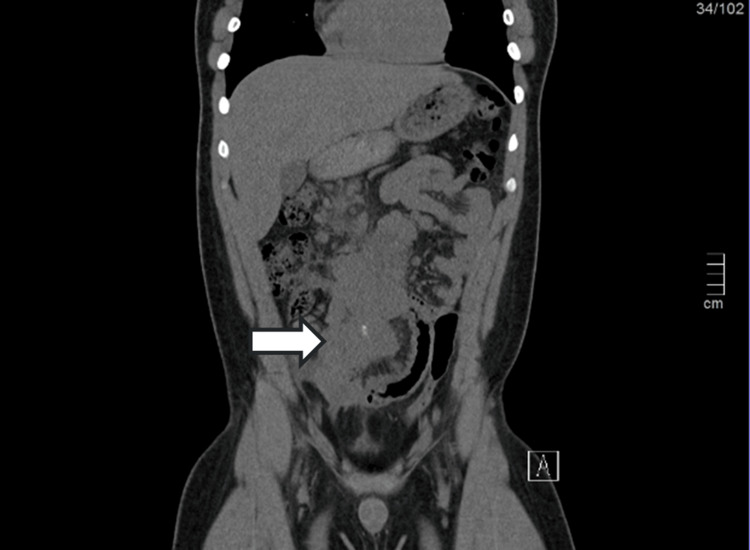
Abdominopelvic CT showing adenopathic conglomerate (front view)
